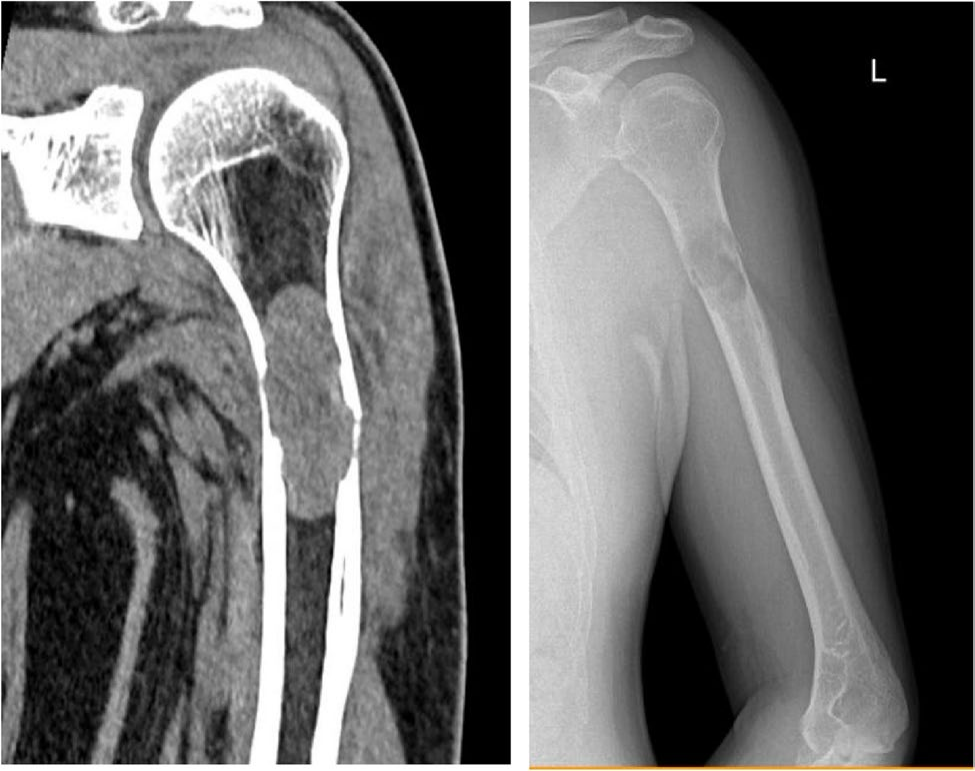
CT(L) and X-ray(R) scans show the left humerus upper segment shows an elongated oval-shaped low-density shadow, with adjacent cortical thinning and fracture
